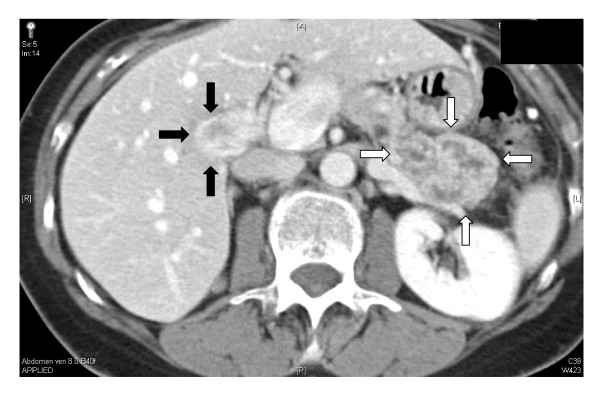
CT scan showing a pancreatic body mass and tail mass (white arrows) with portal vein thrombus (black arrows).
